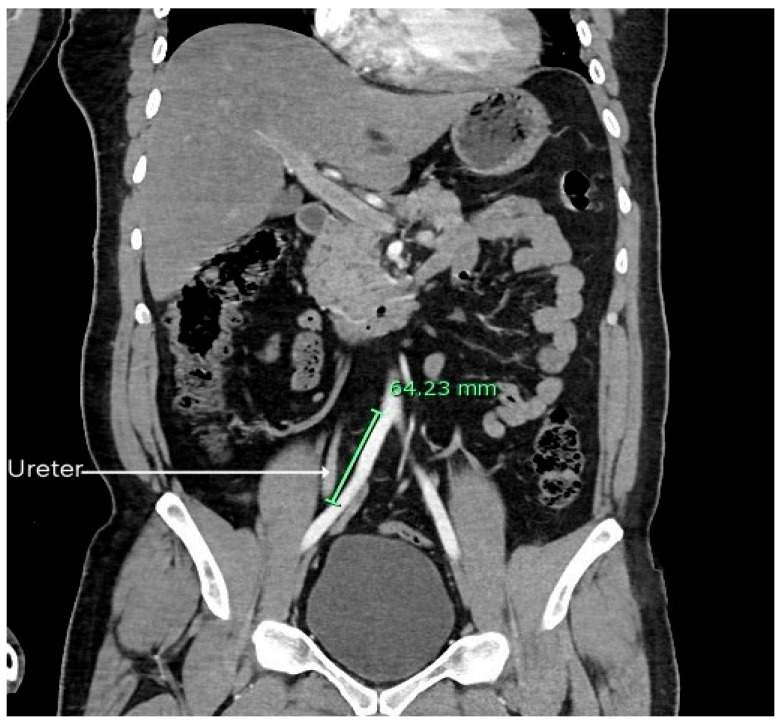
CT scan showing the measured distance between the AA bifurcation and the point where the left ureter crossed the left iliac vessels.
